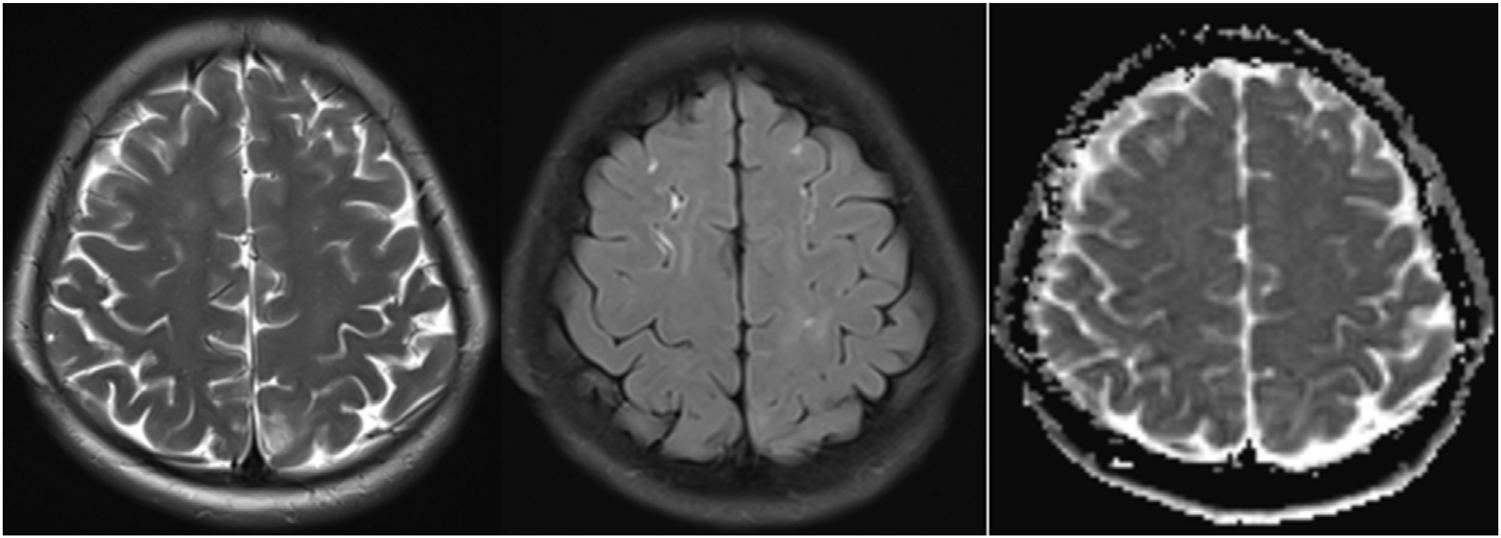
MRI findings after 2 weeks of treatment in AIP: Significant reduction in hyperintense lesions on T2-weighted, FLAIR sequences and ADC map.
